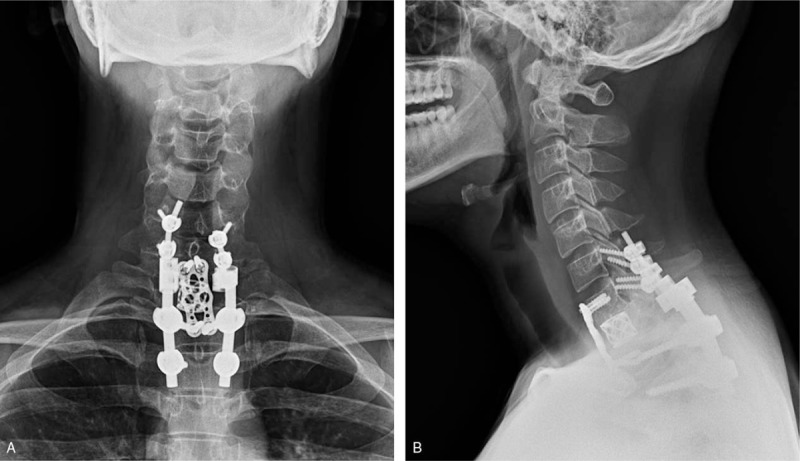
Post-operative X-ray image. (A) and (B) AP and lateral X-ray image showed anterior interbody fusion with titanium graft placement and cervical plating from C7 to T2. And posterior screw fixation was also performed from C6 to T3.
